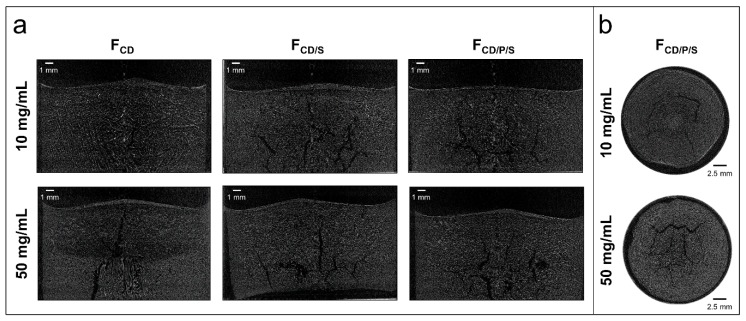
Internal cake structure. Representative µ-CT images for 10 and 50 mg/mL formulations freeze-dried with C5 showing (a) internal cake structure through a vertical cross section of F_CD_, F_CD/S_, and F_CD/P/S_, and (b) cake structure at the bottom of the vial through a horizontal cross section of F_CD/P/S_.
